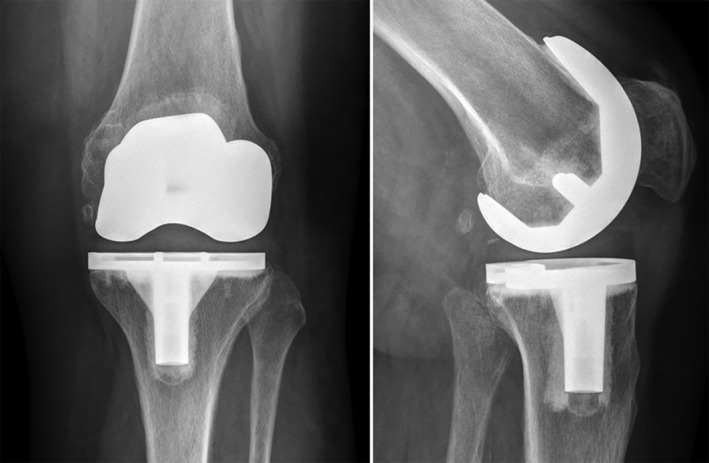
The radiograph shows the x‐ray images of the left knee of a 72‐year‐old female patient at 1‐year follow‐up demonstrating a well‐aligned implant position.
